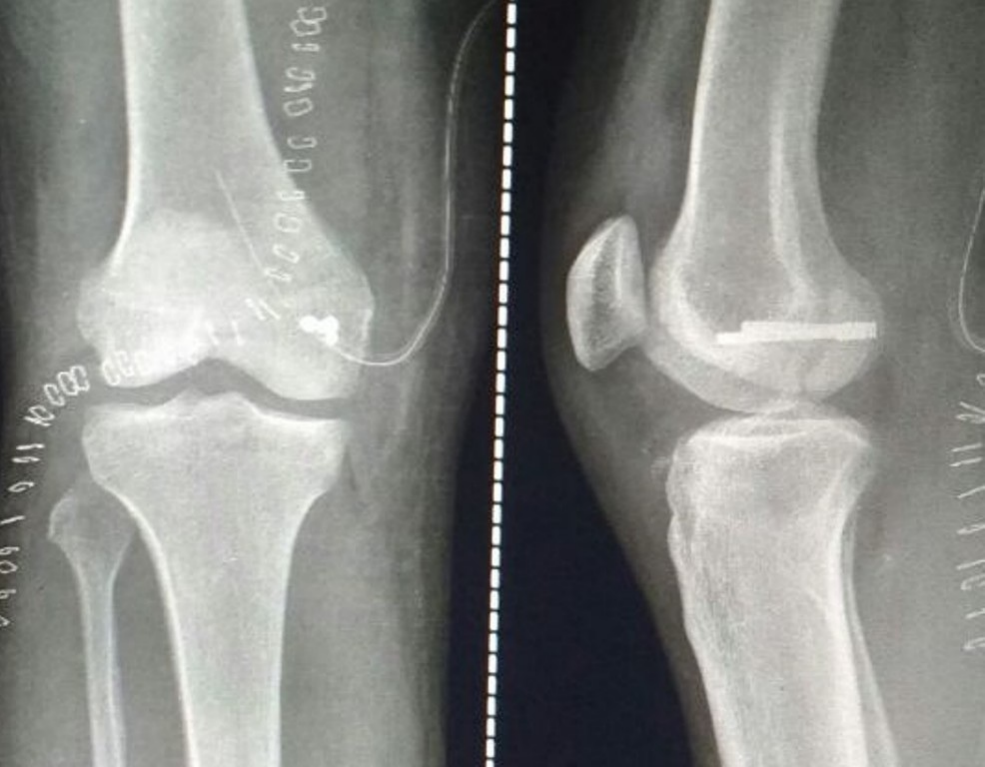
The post-op X-ray images of the anteroposterior and lateral views showing the Hoffa type II(C) fracture fixed with two non-parallel headless Herbert screws after reduction of the fracture.
